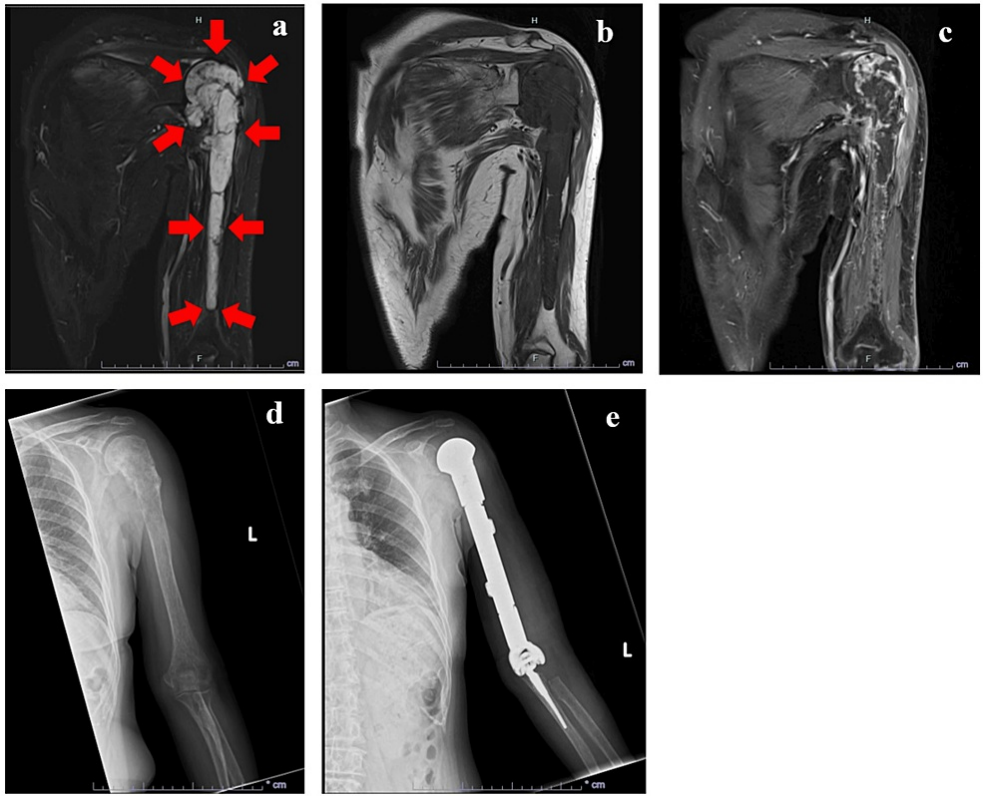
MRI and X-ray images of the left humerus STIR (a), T1-weighted (b), and T1-weighted contrast-enhanced (c) images before surgery and X-ray images before (d) and after surgery (e) are shown. MRI, magnetic resonance imaging; STIR, short τ inversion recovery Arrows indicate the tumor observed from the head of the humerus to the distal four-fifths of the diaphysis.
